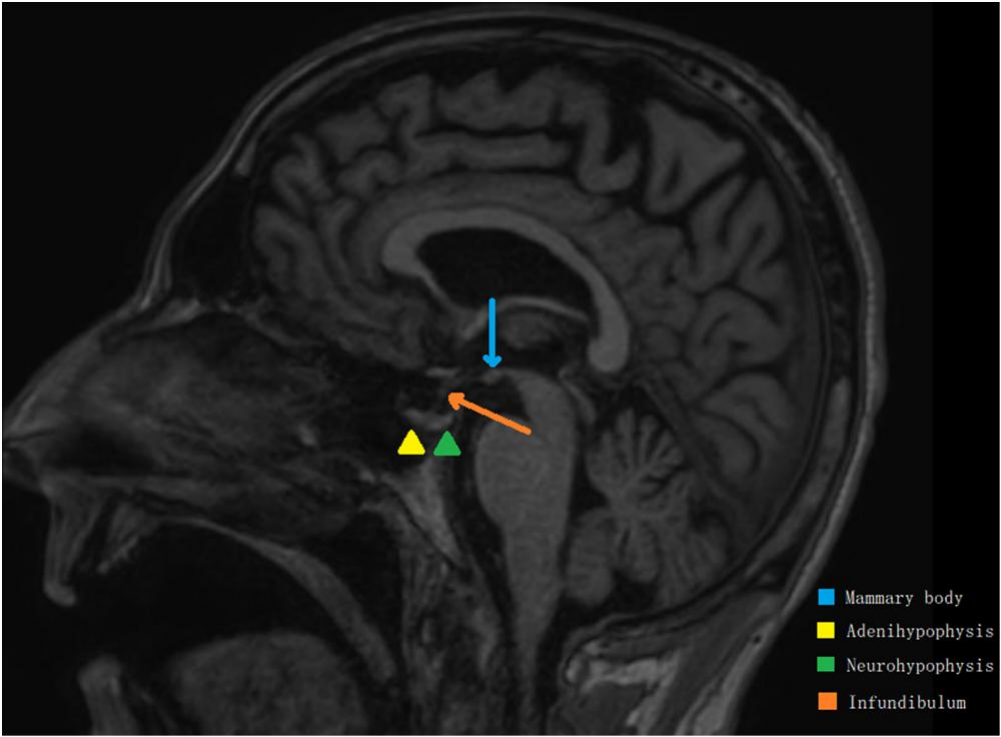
A sagittal magnetic resonance imaging of the pituitary gland showing a normal anatomy with no overt swelling, injury, or mass.
